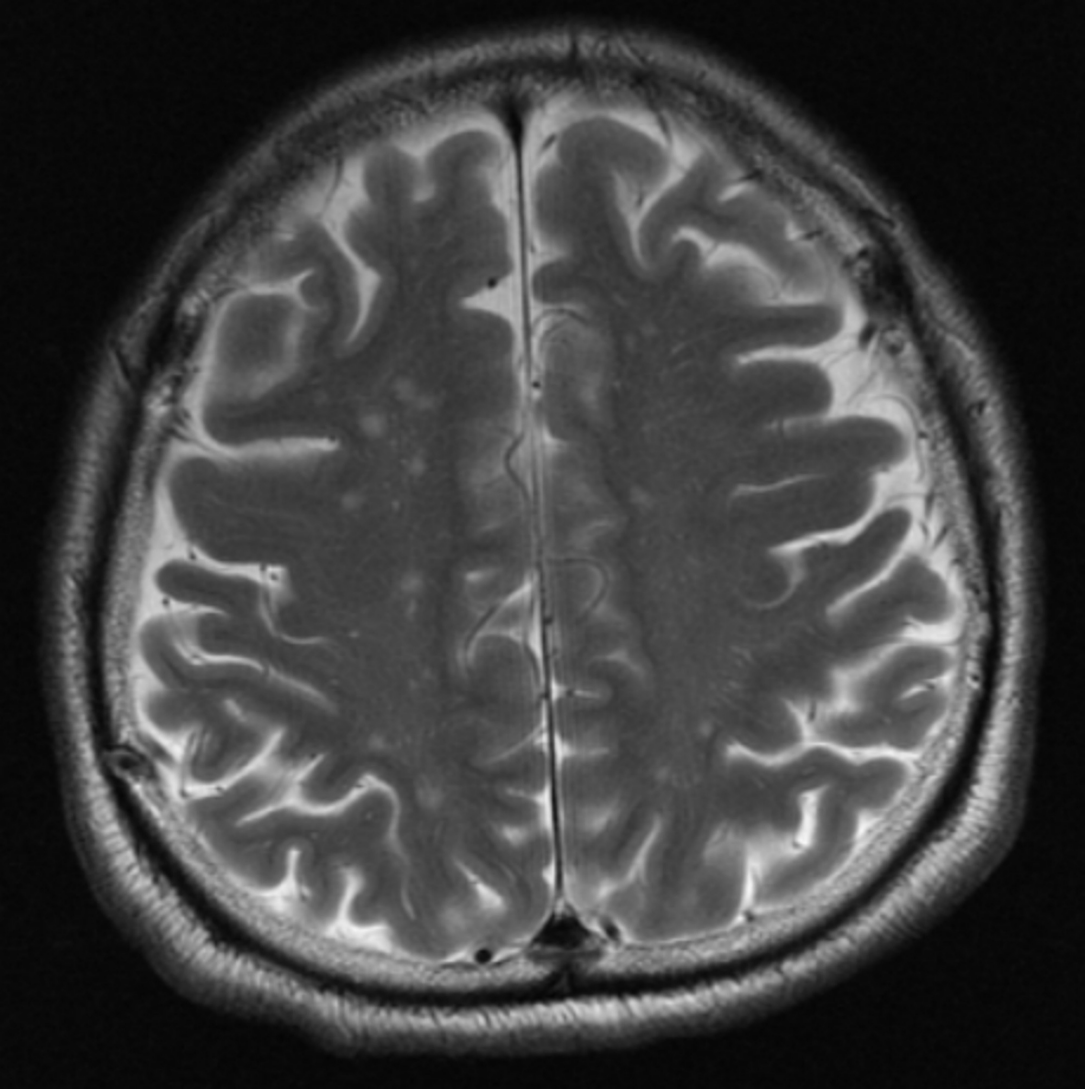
A magnetic resonance imaging scan of the brain, which was done at 17 hours after the index event of bilateral blurring of vision, revealed no evidence of intracranial bleed.
